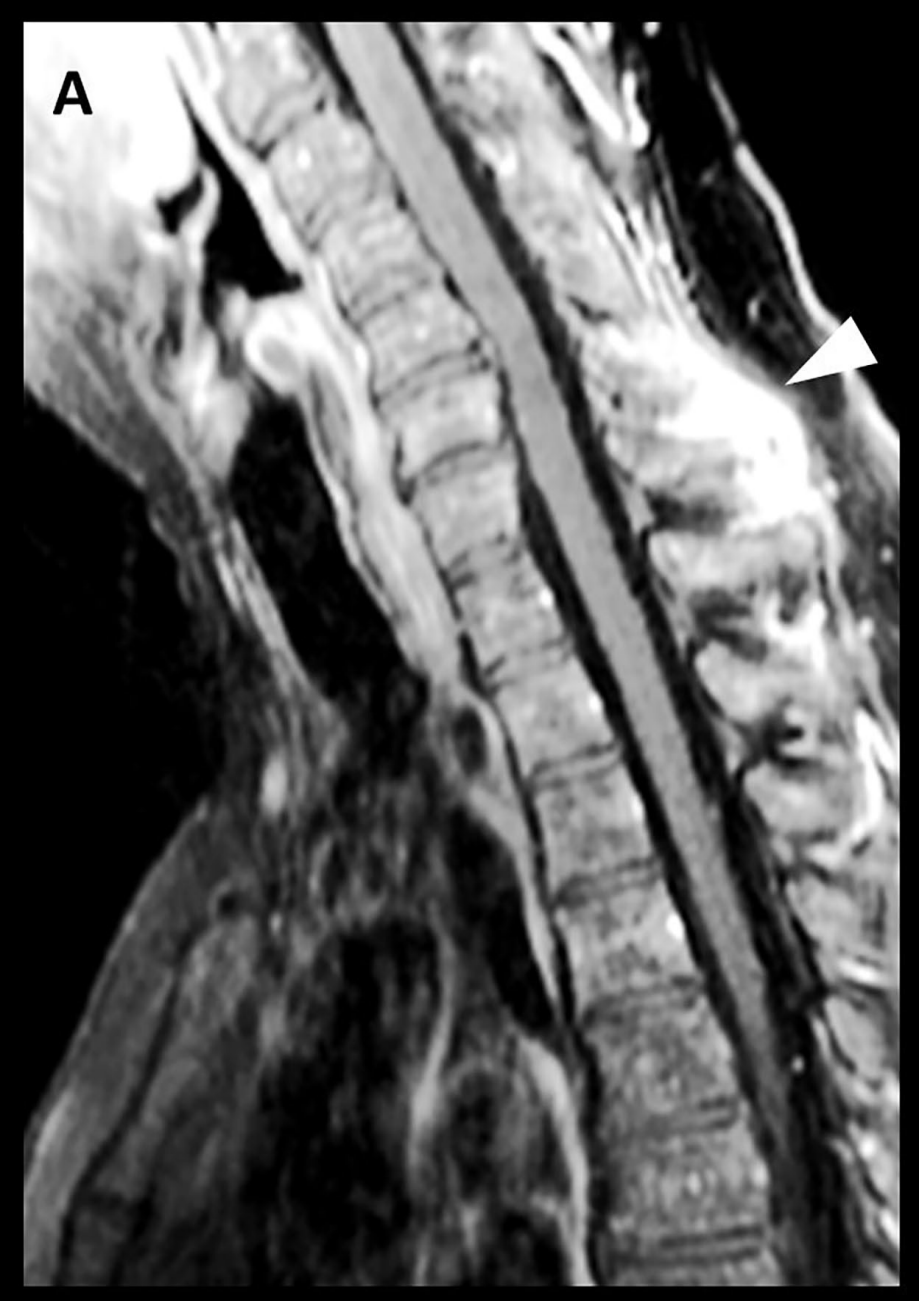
Magnetic resonance imaging (MRI) with contrast of the cervical spine, sagittal view (A) Whole-spine MRI, this time with contrast, clearly revealed an increased contrast enhancement around the C7 spinous process, suggestive of a possible soft-tissue inflammatory process or an abscess formation (white arrowhead).
